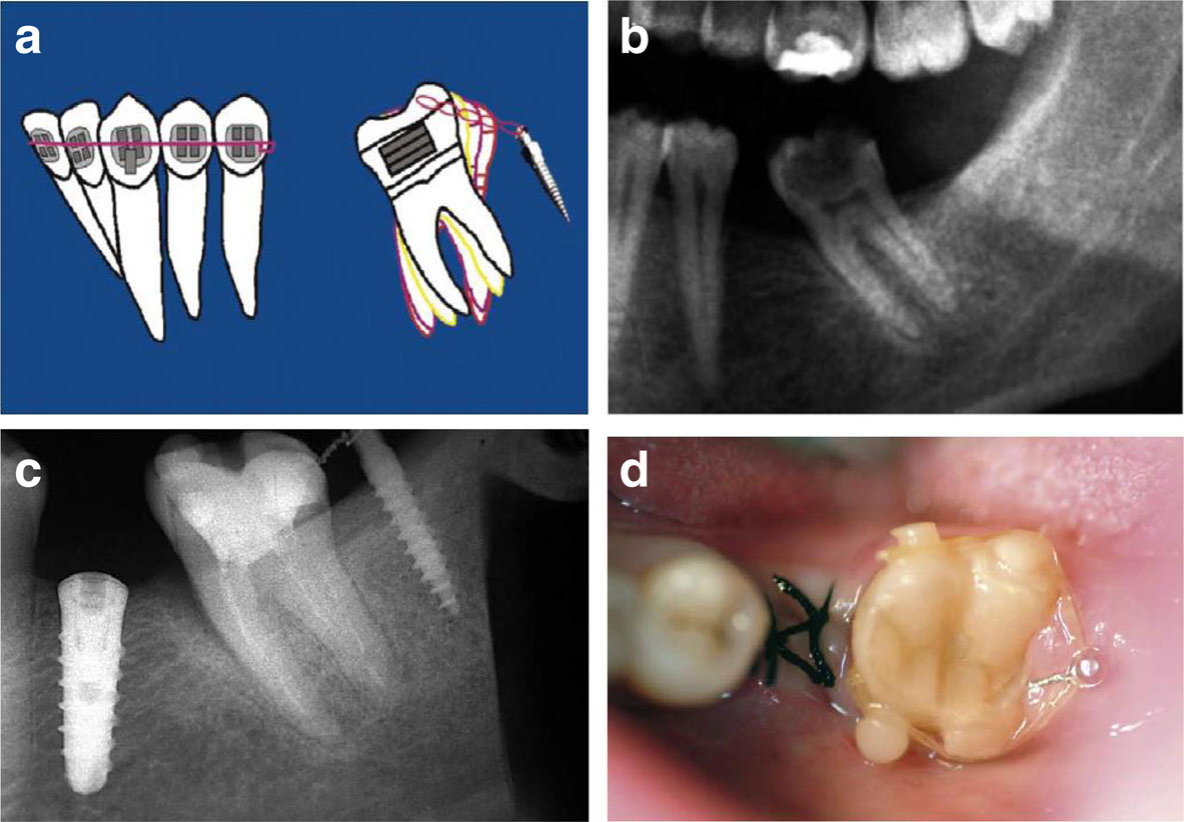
Uprighting of #37 with a miniscrew implant and elastomeric chain. a A miniscrew positioned in the retromolar area with an elastomeric chain between the screw and the molar; uncontrolled tipping. b Initial radiograph at the area of #37. c Final radiograph after the uprighting of #37, with the miniscrew placed distally and an implant in the site of #36. d Occlusal view of uprighted #37. (From Musilli et al. [2], with kind permission of Progress in Orthodontics)
